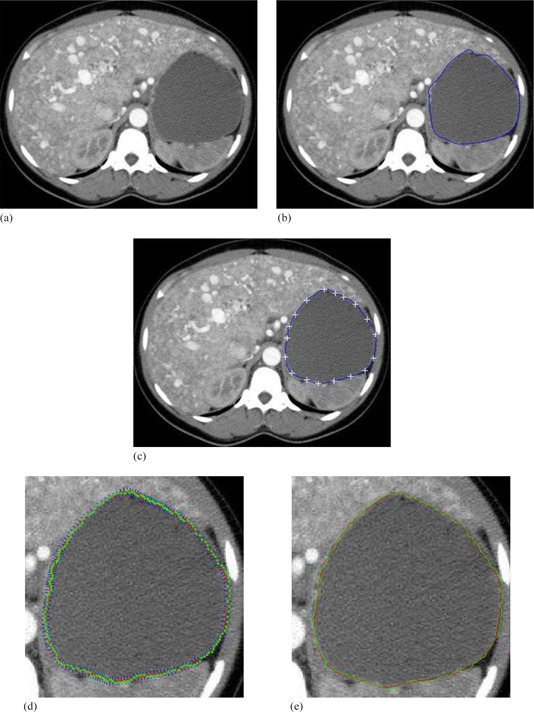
Contrast‐enhanced axial CT scans through liver:^(34)^ (a) original image; (b) initial manual delineation; (c) manual revision with control points; (d) curve refinement; (e) the manual revised contour (red) and the curve refined contour (green).
